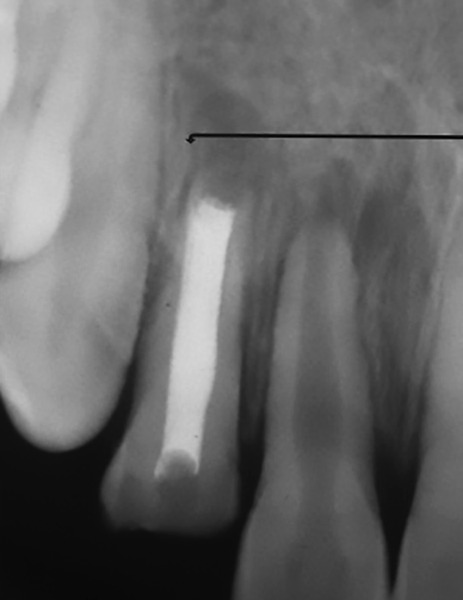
Intraoral periapical radiograph with an arrow showing retrograde filling done using zirconomer-reinforced glass ionomer cement and removal of root apex
